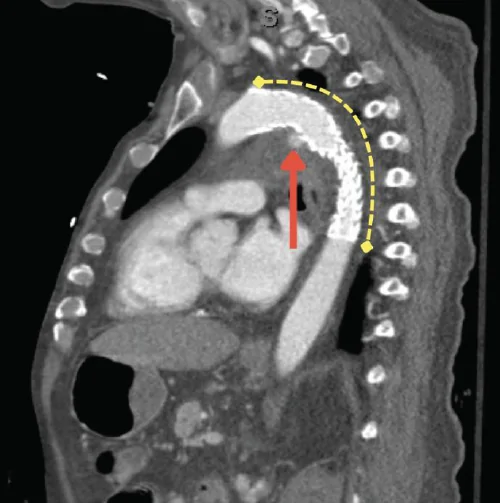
Computed tomography angiogram of the chest demonstrating the length of the endograft (broken yellow line) and endoleak arising from the anterior/inferior aspect of interval endograft repair of the aortic arch (red arrow).
